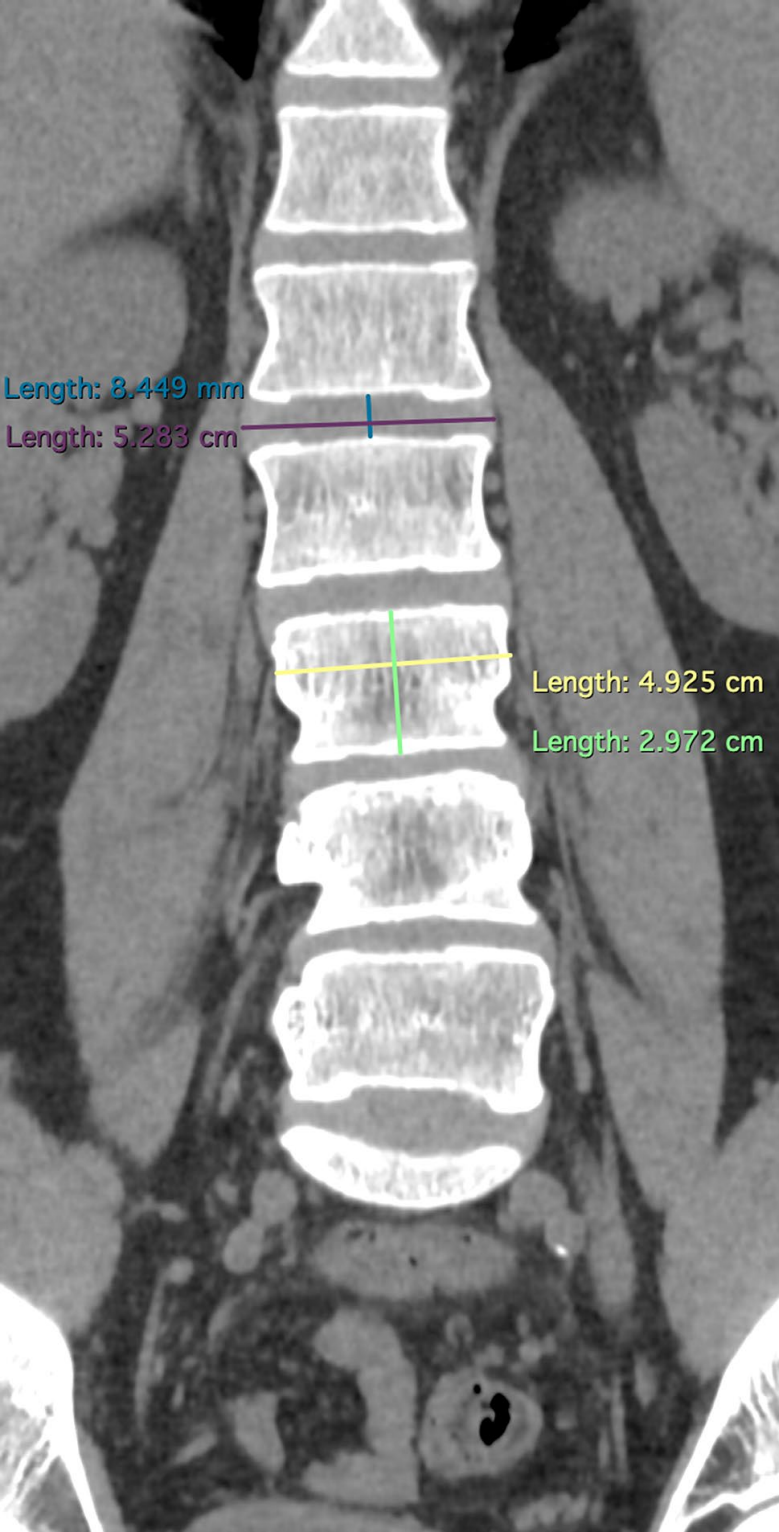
Lumbar spine computed tomography (coronal) of verterbra’s and disc’s height and width
